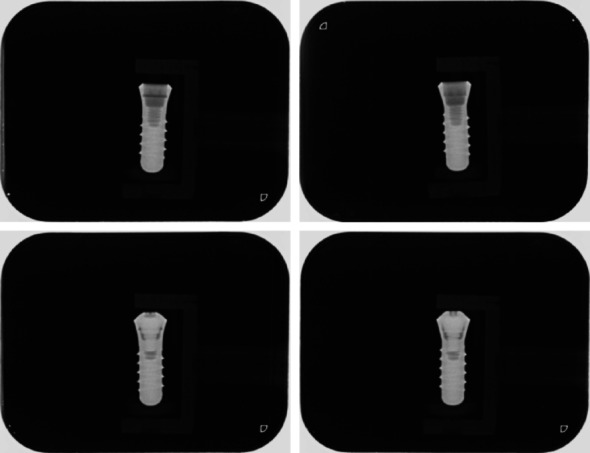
Representative radiographs of the implants included in the dataset; Top left: Straumann® implant without screw; Bottom left: Straumann® implant with screw; Top right: Meisinger® implant without screw; Bottom right: Meisinger® implant with screw
